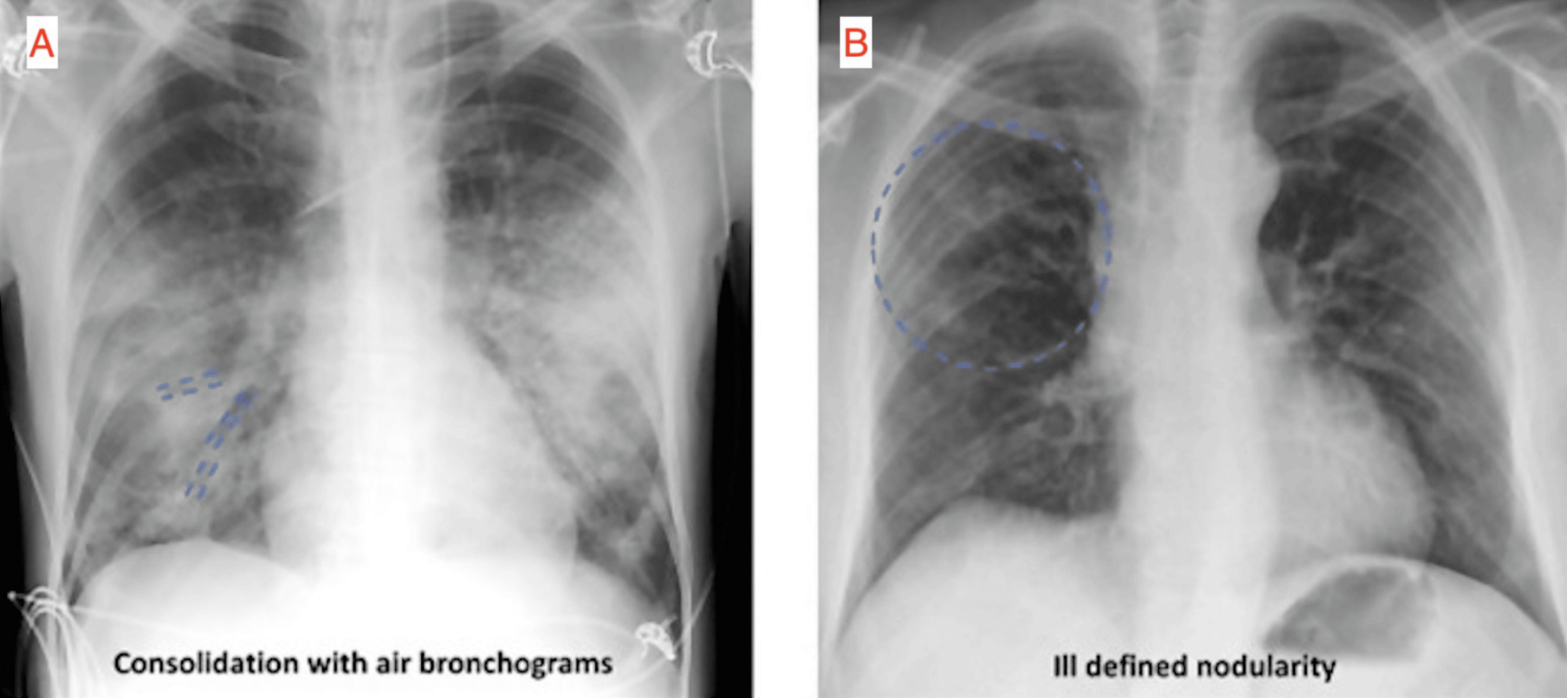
Representative CXR images of COVID-19 pneumonia CXR: chest x-ray; AP: anteroposterior (A) AP CXR shows consolidation with air bronchograms in the right lung. (B) AP CXR shows ill-defined nodularity of the right lung Image adapted and reproduced from Bahra et al. [10] under the CC BY 4.0 license
